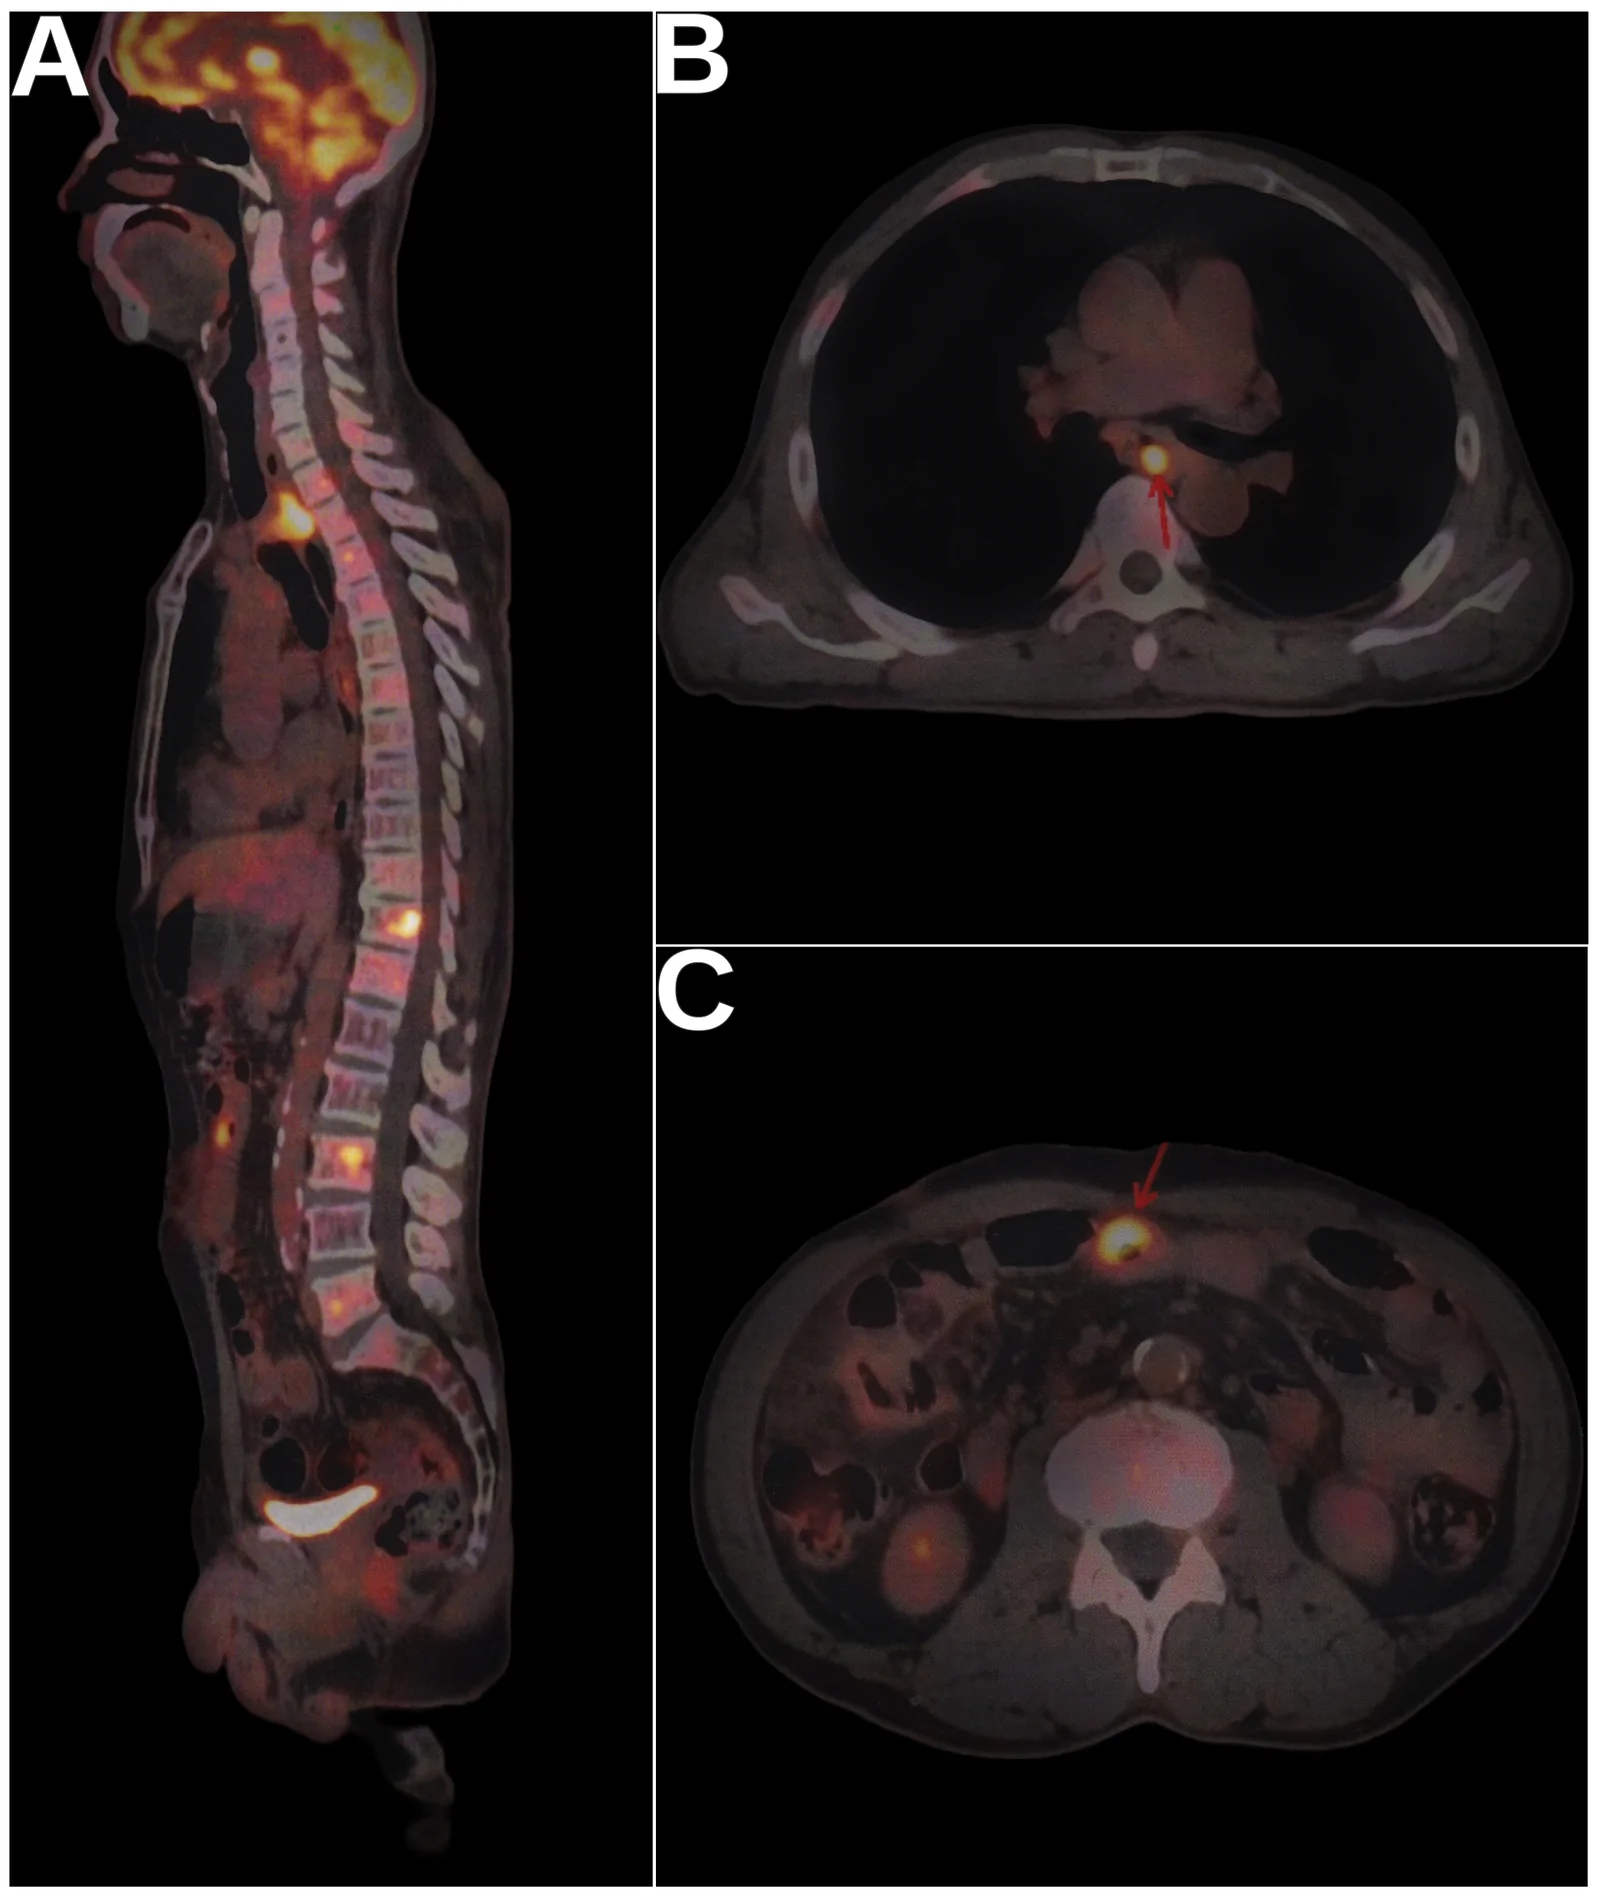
Whole-body PET/CT for primary tumor localization and staging (A) Axial fused ^18^F-FDG PET/CT at T6 level showing a mid-esophageal soft-tissue nodule (0.8×0.9×2.2 cm) with increased uptake (SUV_max_=6.0), consistent with primary esophageal squamous cell carcinoma. (B) Axial fused PET/CT at the jejunal anastomosis showing circumferential thickening (1.7×1.4 cm) with mild uptake (SUV_max_=5.3), likely post-surgical inflammation. (C) Maximum intensity projection (MIP) image revealing widespread systemic metastases: hypermetabolic supraclavicular, hilar, mediastinal, subdiaphragmatic and retroperitoneal lymph nodes (SUV_max_=5.9); diffuse osseous metastases (scapula, ribs, vertebrae, pelvis, femurs); and a suspicious hepatic lesion in segment S8 (SUV_max_=2.2). (All acquired August 25, 2022.).
